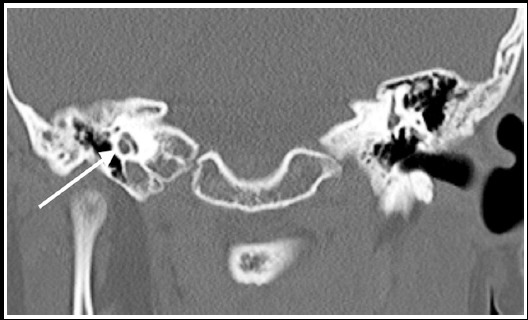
Thin-slice coronal computed tomographic image of a sample case with normal inner ear anatomy obtained before cochlear implantation surgery (Cochlea has 2.5 spirals, vestibule and other structures are natural).
